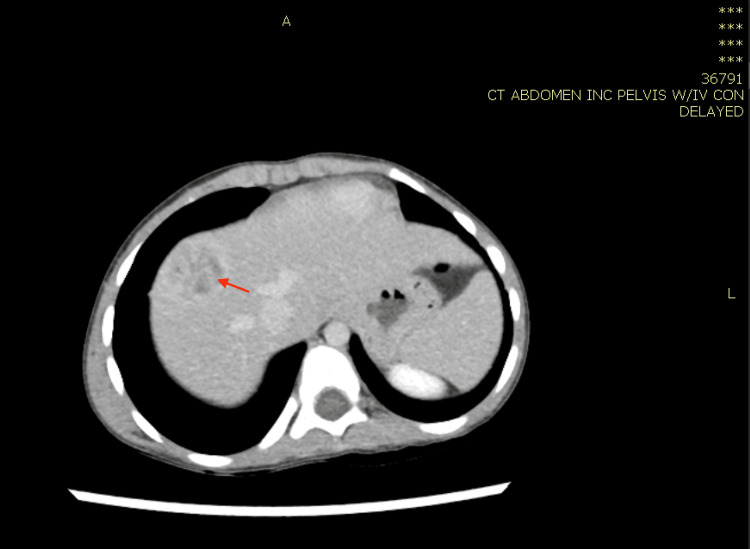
Computed tomography scan of the abdomen with IV contrast showing enlarged liver with large, ill-defined mass lesion of the medial segment of the left hepatic lobe measuring approximately 6 cm, mildly hypodense to isodose (red arrow).
